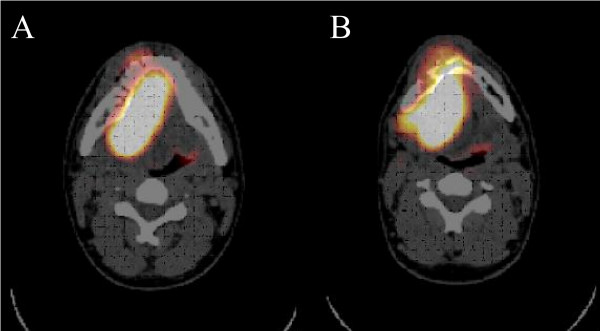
a, b: Radionuclid (FDG) enhancement in the right floor of the mouth expanding over the midline.  No further enhancement in the whole body scan visible.
